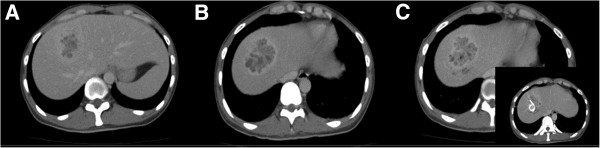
Computed tomography images of the lesion. A) Cystic heterogeneous lesion in segment 8 of the right liver lobe, with a partly liquefied component. B) Axial images showing a rim-enhancing lesion suggestive of residual liver abscess after drainage and vancomycin treatment. C) Axial images showing a rim-enhancing lesion suggestive of residual liver abscess after drainage with a larger tube and teicoplanin treatment.
